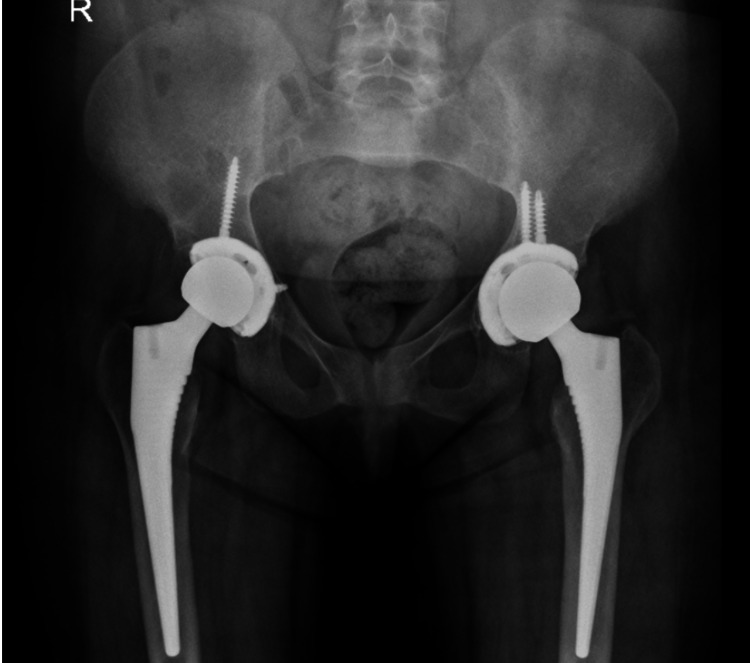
Radiograph of the pelvis seven months after the second total hip arthroplasty with no unusual features or major complications evident.
